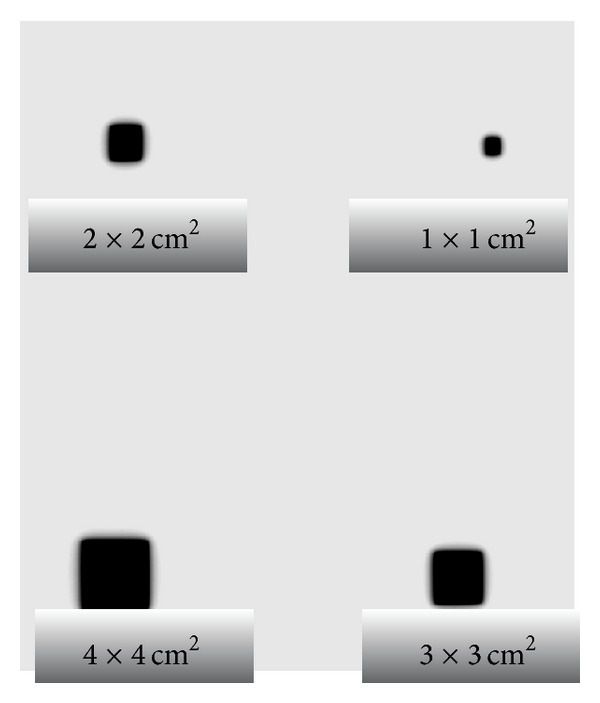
Radiographic film exposed to 15 MV for various fields.
